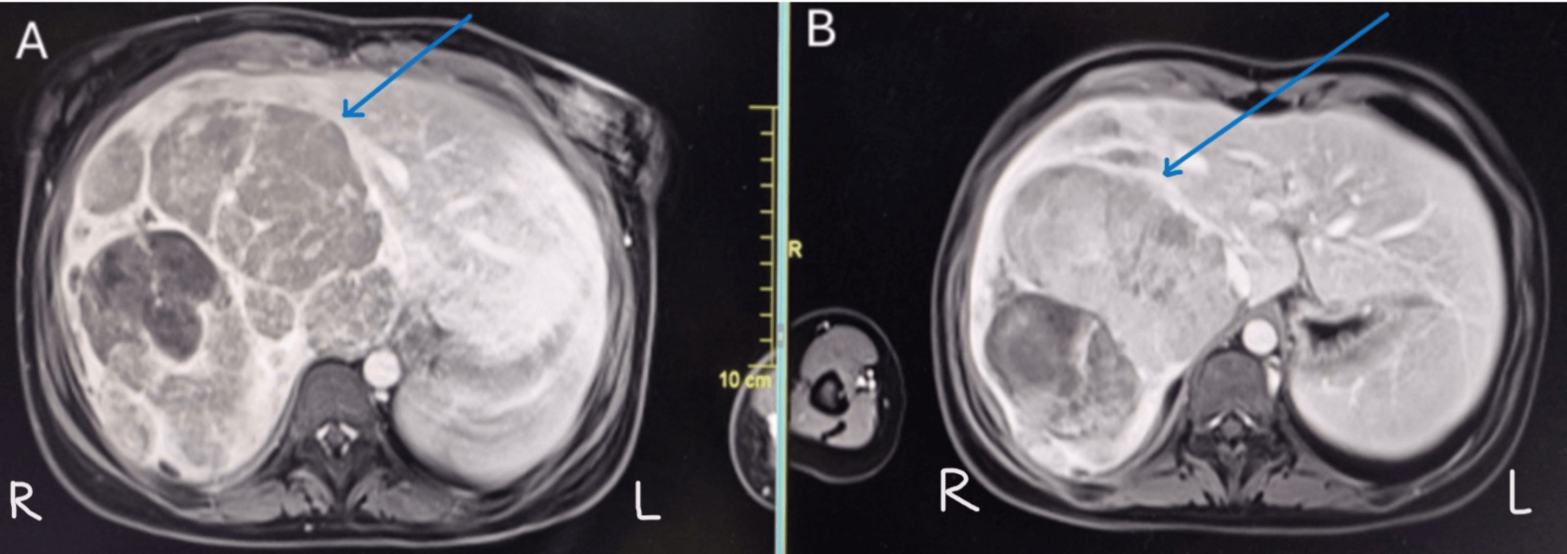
(A) Contrast-enhanced MRI of the liver showing a large, heterogeneous right lobe mass with restricted diffusion and enhancing solid components (arrow); (B) post-neoadjuvant imaging demonstrating normal left lobe of the liver and mild interval tumour shrinkage with preserved left lobe, corresponding to PRETEXT III staging (arrow).
